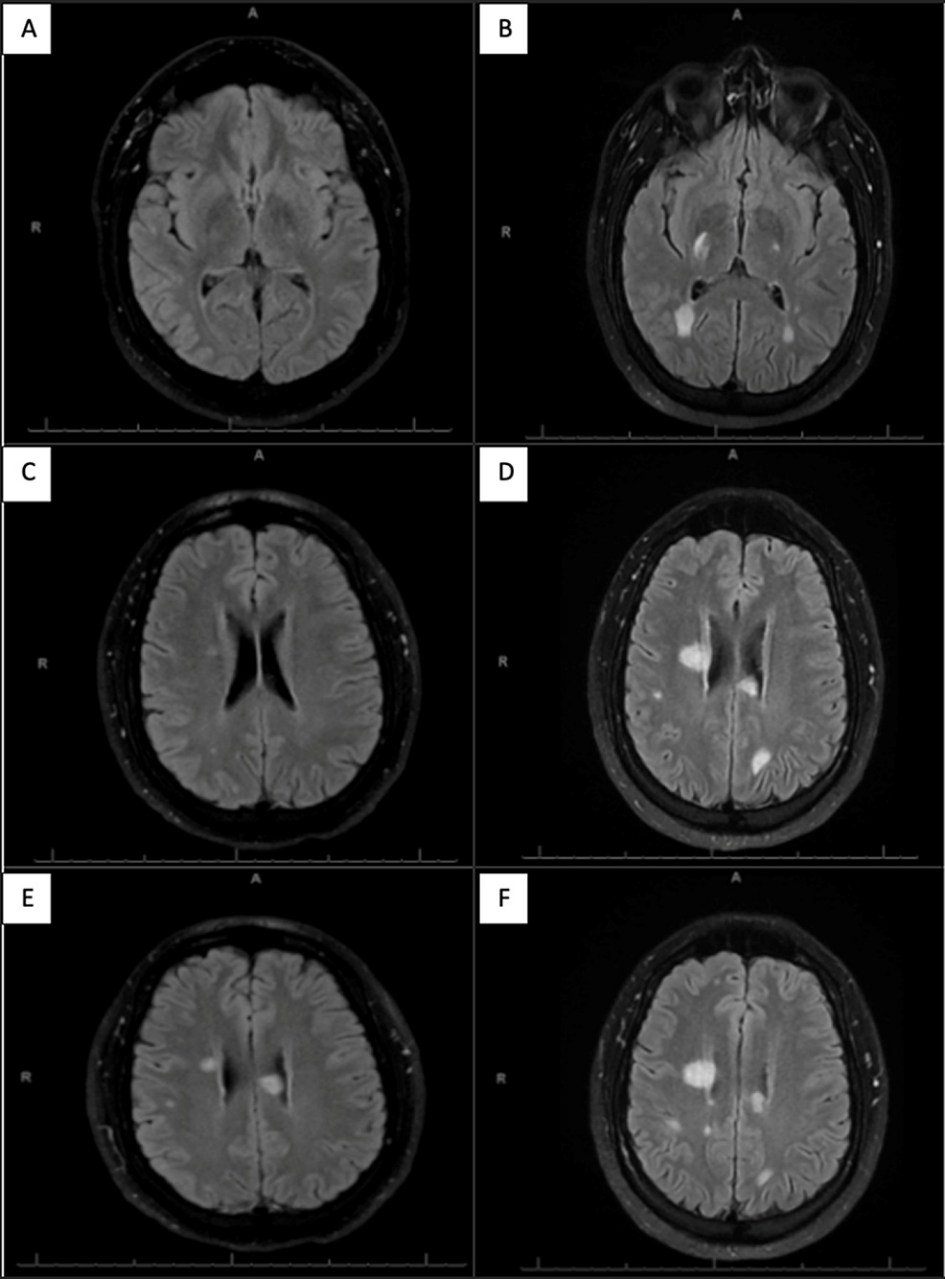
T2 Axial MRI Images T2 axial MRI images were taken on 5/7/21 at an outside hospital (A,C,E) and 5/31/2021 at our hospital (B,D,F) which show increased contrast uptake in focal white matter lesions and progressive disease. Figure A demonstrates an absence of plaque burden at this level during acute presentation and figure B shows four new lesions at the same level. Figure C shows a small right periventricular plaque while Figure D demonstrates a significant enlargement of previous plaque and development of three additional lesions. Figure E reveals bilateral periventricular plaques and a small right peripheral lesion. Figure F demonstrates a significant enlargement of plaques at the same level and three to four new lesions.
